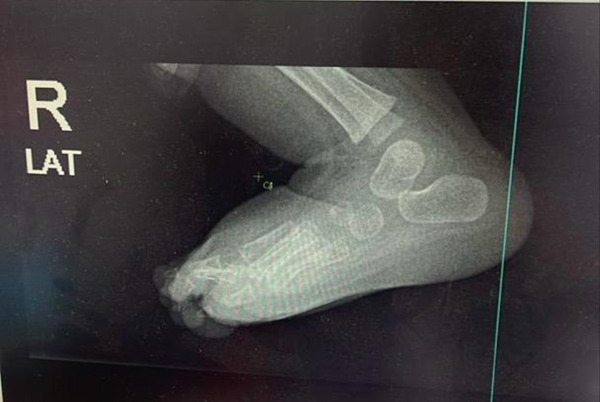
A lateral view of the plain radiograph of the right foot.
